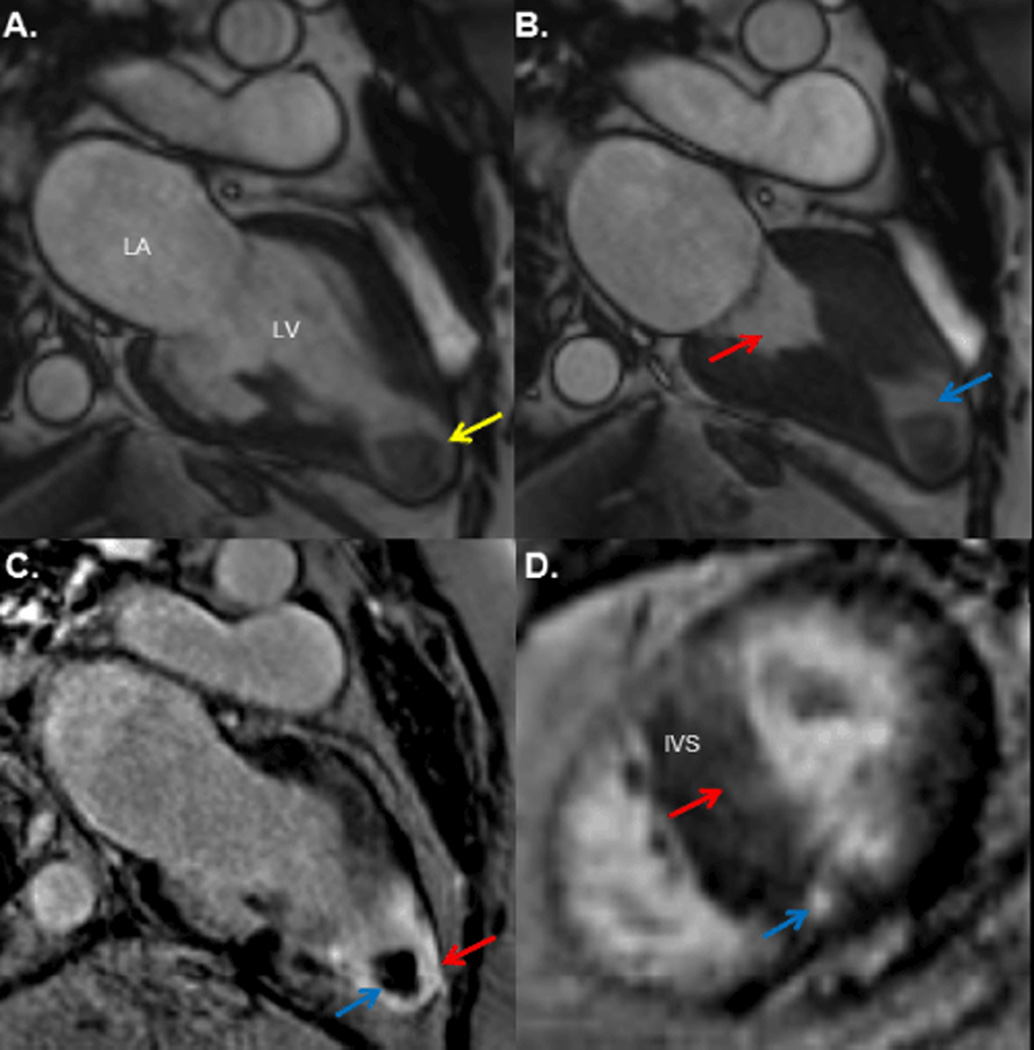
Cardiovascular magnetic resonance imaging. A. Steady-state free precession image of the two-chamber view at end diastole demonstrating a spade-shaped left ventricular cavity with mid ventricular hypertrophy and aneurysmal apex containing a mass (arrow) measuring 24 × 20 mm. B. The corresponding view at end systole demonstrates midventricular cavity obliteration resulting in separate apical (blue arrow) and ventricular chambers (red arrow). C. Late gadolinium enhancement imaging shows transmural fibrosis (bright) of the myocardium (red arrow). The periphery of the apical mass enhances (blue arrow) while the core remains hypointense (black), consistent with thrombus. D. Late gadolinium enhancement imaging of a short-axis mid ventricular view also demonstrates inferoseptal right ventricular insertion fibrosis (blue arrow) and patchy fibrosis of the interventricular septum (red arrow), consistent with hypertrophic cardiomyopathy. IVS: Interventricular septum; LA: Left atrium; LV: Left ventricle
